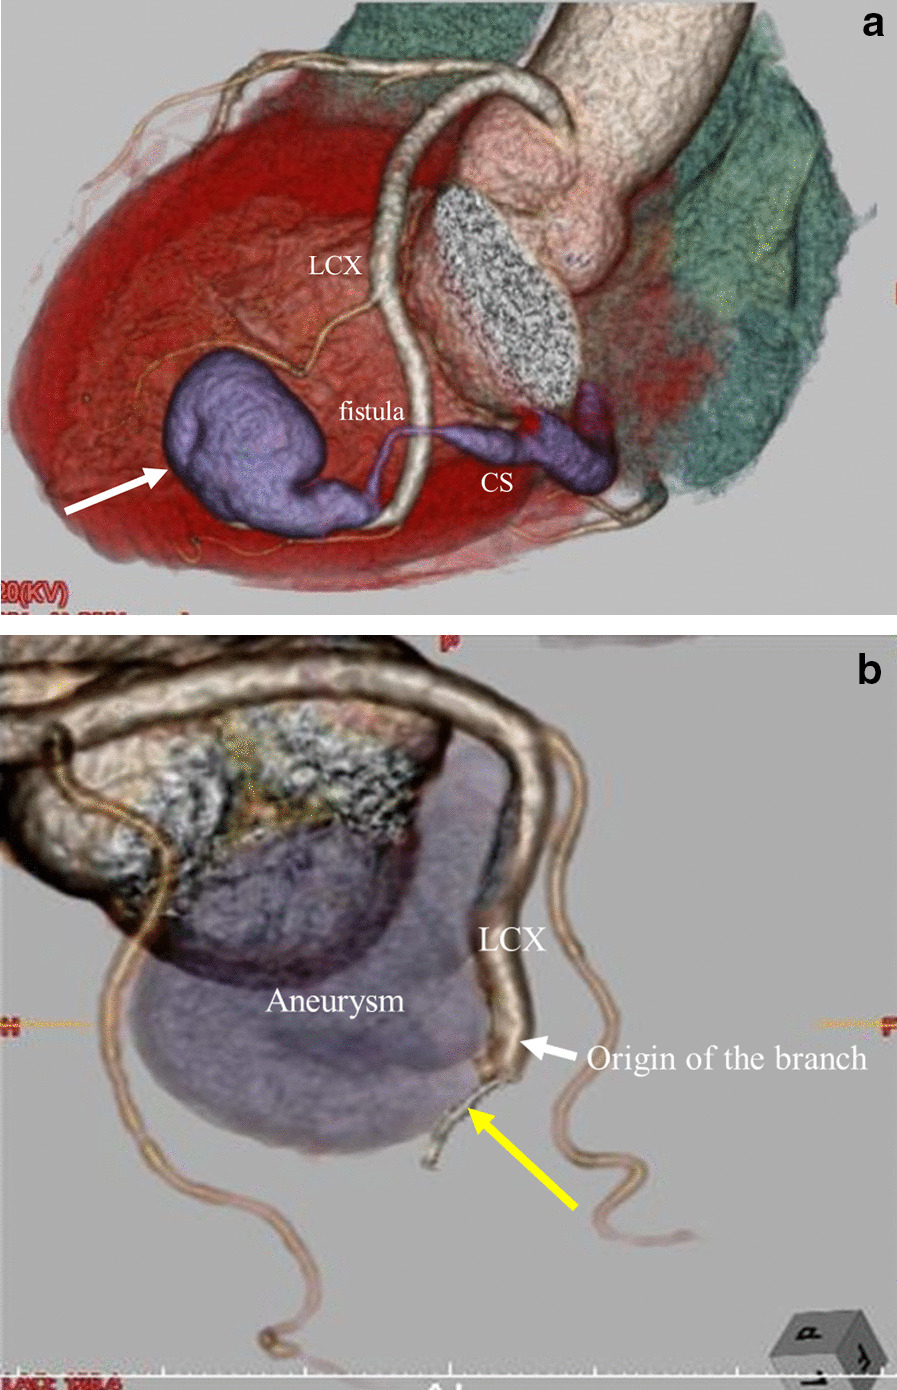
a Preoperative coronary artery computed tomography revealed a giant coronary branch aneurysm originating from near the terminal portion of the main LCX trunk with a fistula draining into the CS (white arrow). b The end of the main LCX was very thin (yellow arrow). CS, coronary sinus; LCX, left circumflex artery
